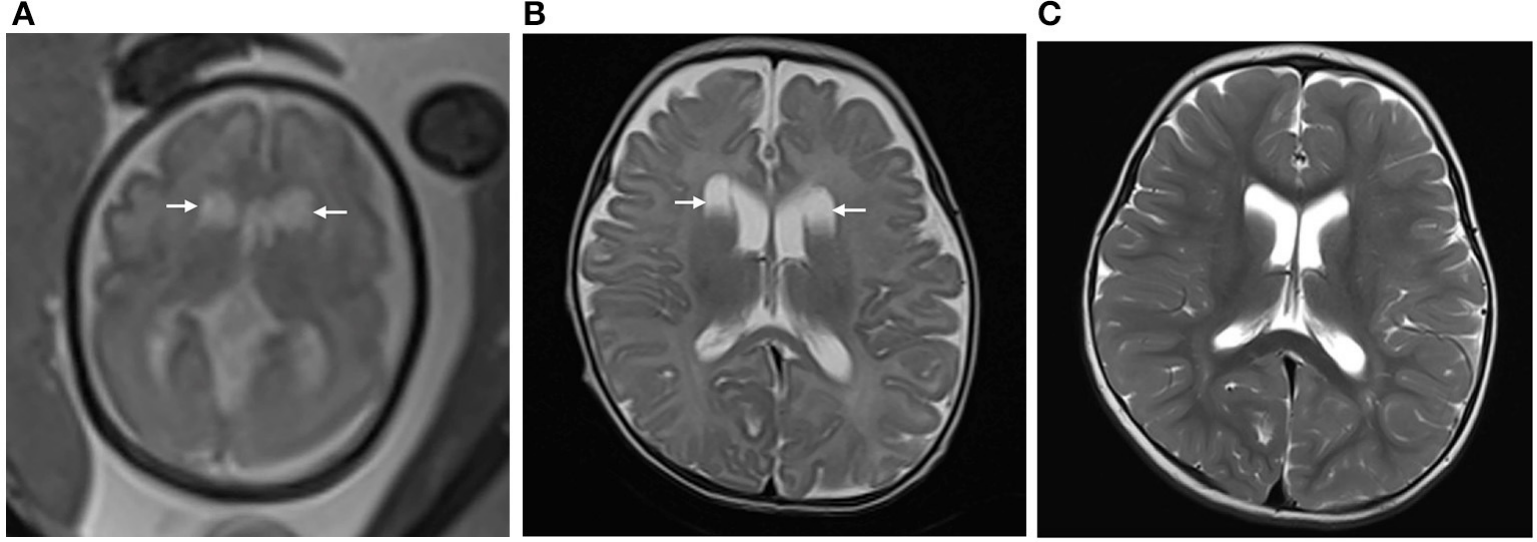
(A) Pregnant woman, 29 years old, with a fetus of 30 weeks' gestation. Fetal brain MRI shows bilateral isolated periventricular pseudocyst (PVPC) located at the external angle of the lateral ventricles (white arrow). The anteroposterior diameter is 1.5 and 1.6 cm, respectively; (B) 40 days after birth, the cyst sizes show no significant change; and (C) At the age of 18 months, the PVPCs disappeared, and the Gesell Developmental Scale (GDS) test showed the developmental quotient (DQ: Adaptive: 96, Gross Motor: 87, Fine Motor: 89, Language: 96, and Personal-Social: 91) was normal.
